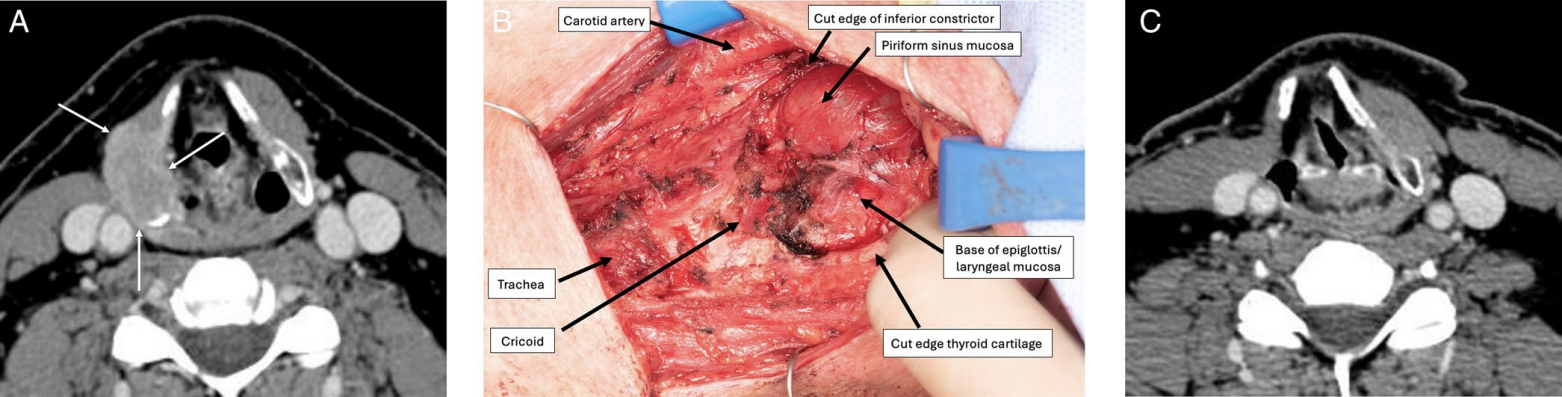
(A) CT neck with contrast delineating 2.8 cm recurrent papillary thyroid carcinoma (arrow) invading the right thyroid cartilage and extending into the paraglottic fat. (B) Intraoperative photograph following extended right hemithyroid cartilage resection (right and partial left), demonstrating surgical defect, with ballooning pharyngeal mucosa and base of epiglottis with associated mucosa. (C) CT neck with contrast 6 months after surgery demonstrating right thyroid cartilage defect and dilated right pyriform sinus, without evidence of disease.
